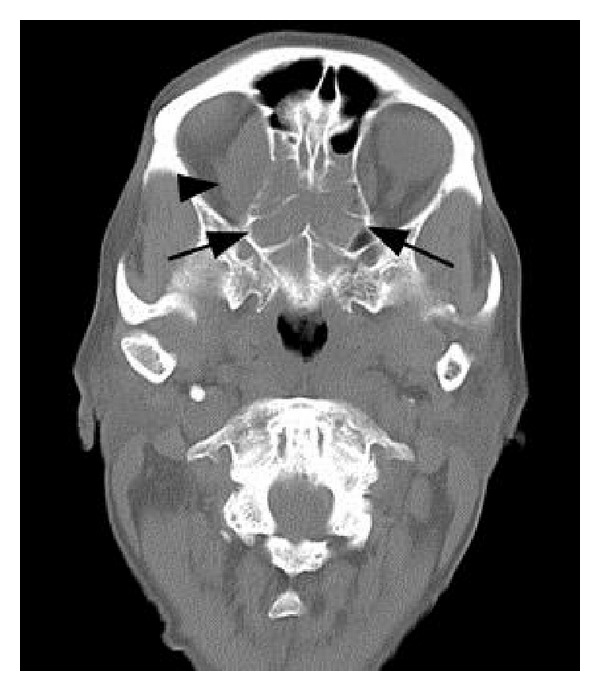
Axial noncontrast brain CT (bone algorithm image) showing a large sinonasal mass involving the ethmoid/sphenoid minuses (arrows) with right orbital extension (arrowhead).
